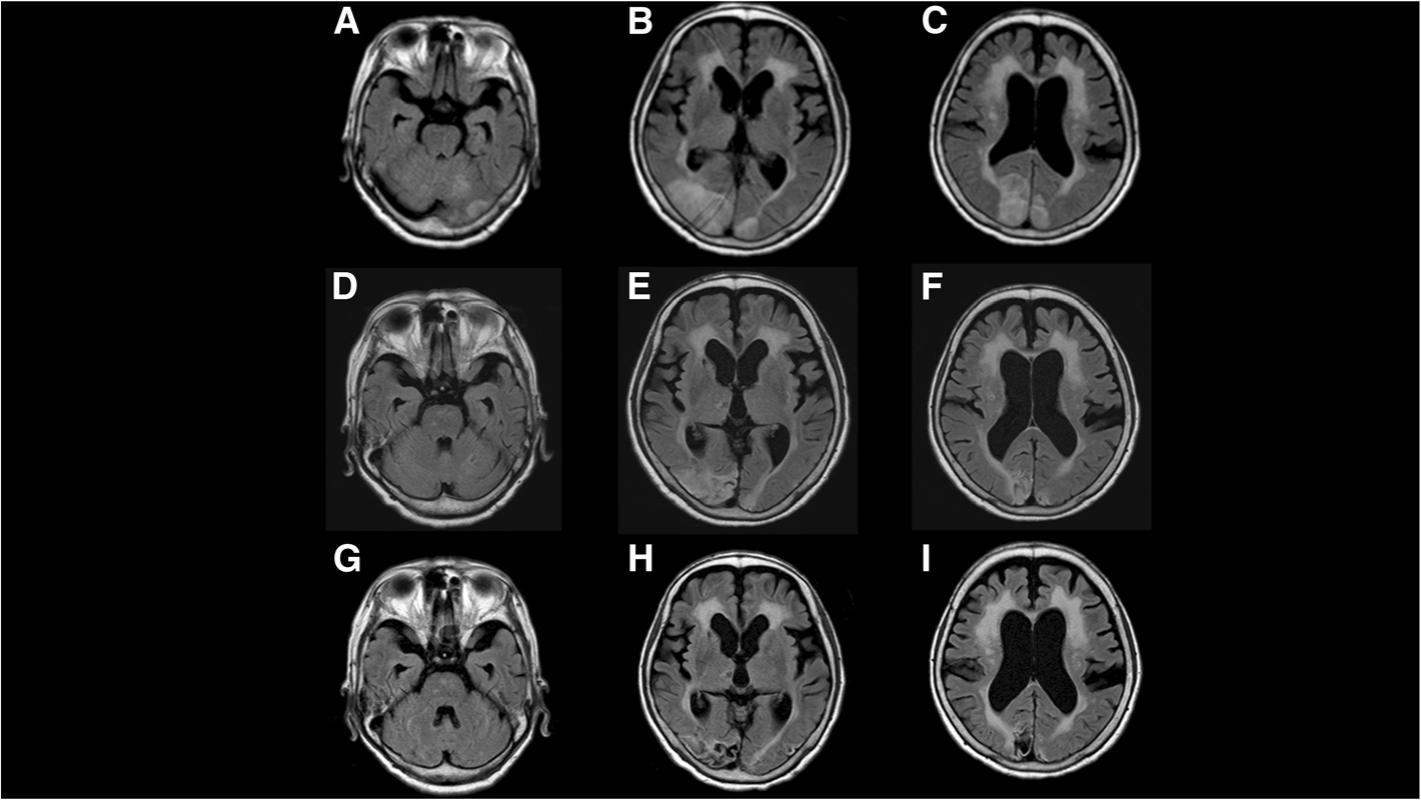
Hyperintense areas on axial fluid-attenuated inversion recovery (FLAIR) image at 2 days after admission (a, b, c) gradually disappear at 2 months after admission (d, e, f) and 4 months after admission (g, h, i). However, at 4 months after admission several cystic changes remained in the cerebellar hemisphere, occipital region, and right thalamus (g, h, i)
